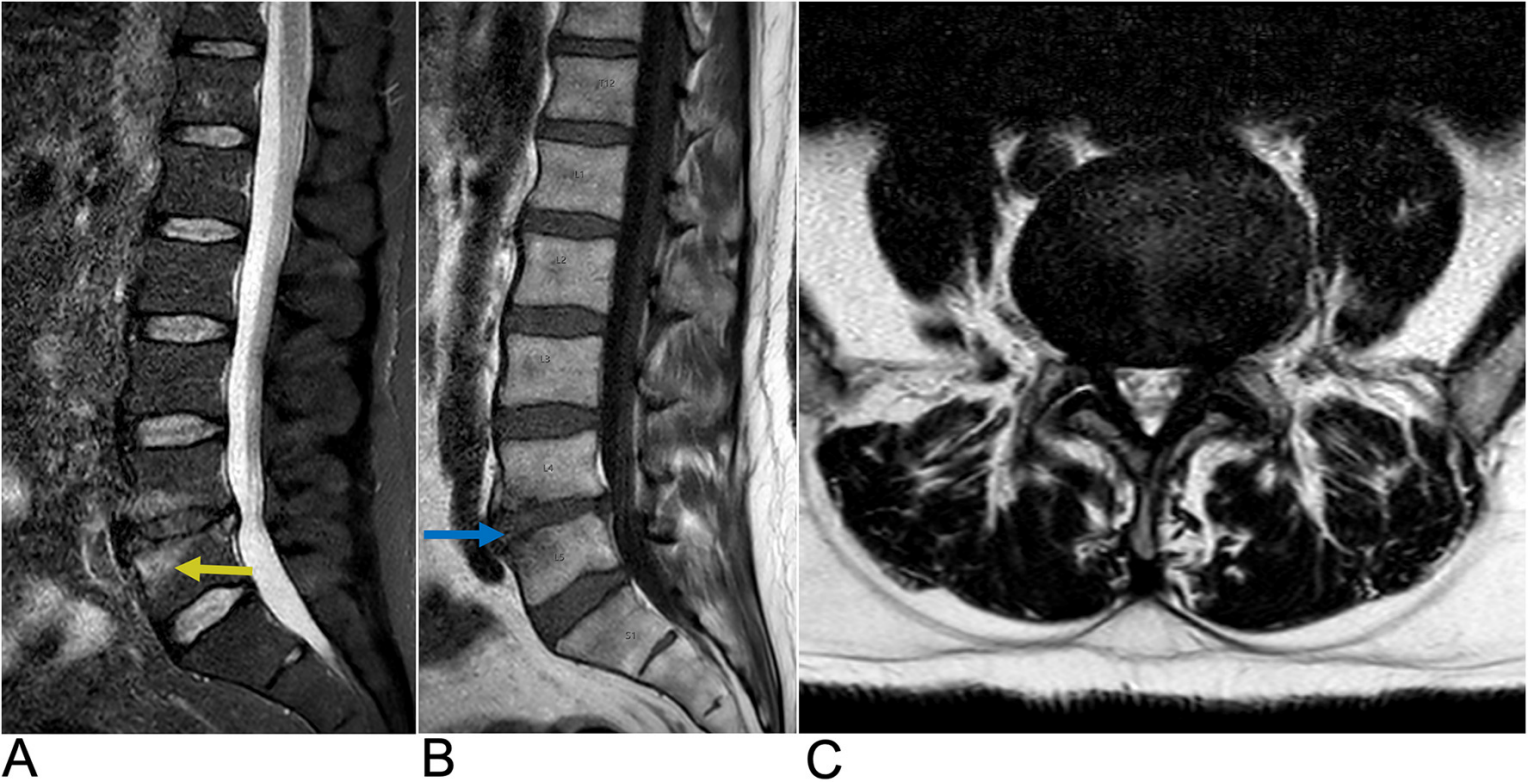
Disc degeneration. Sagittal T2 (A), sagittal T1 (B), and axial T2 (C) MRI demonstrating chronic spondylitic degenerative changes at L4/5 including diffuse disc bulge (blue arrow), loss of intervertebral disc height, and Modic Type 1 endplate degenerative changes (yellow arrow).
